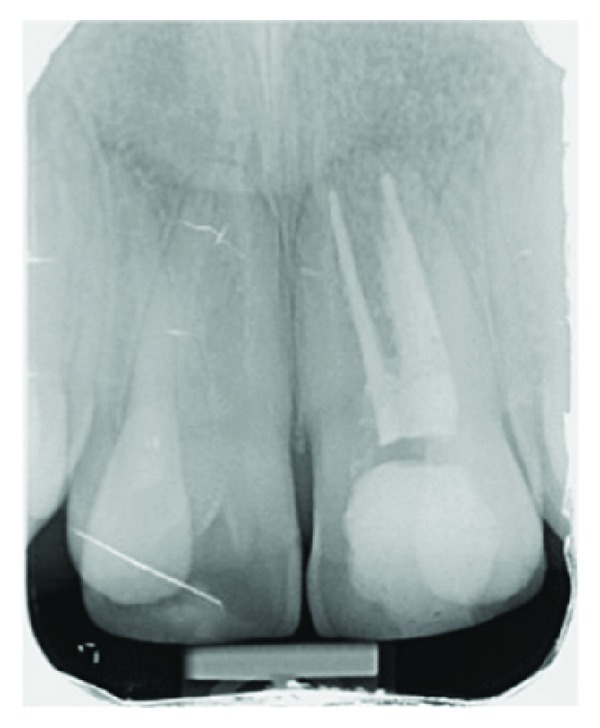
Postoperative final periapical radiograph of the left central incisor.
